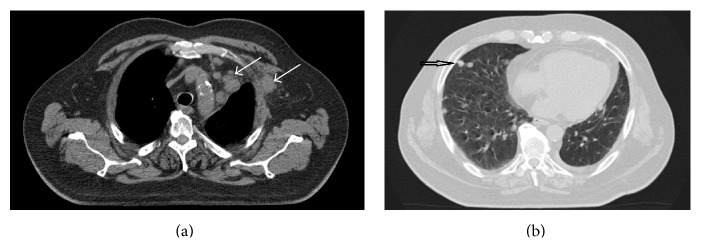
(a) Computed tomography scan of the thorax showing left-sided axillary nodal metastasis and mediastinal lymph node metastases. Status before palliative radiotherapy. (b) Computed tomography scan of the thorax showing right-sided pulmonary metastases (example of the patient's bilateral metastases). During radiotherapy, this region received a cumulative dose of <1 Gy.
